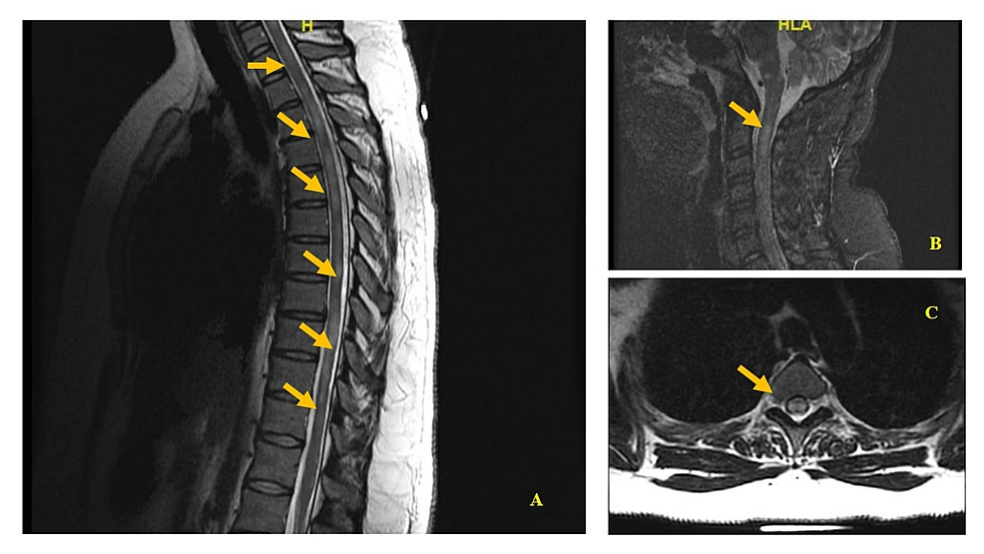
Magnetic resonance imaging of the thoracic spine. (A) and (C) show a plane image of the central spinal cord with a large ill-defined patchy hyperintense signal. (B) Axial T2-weighted cut (arrow) showing a slight expansion of the spinal cord diameter and hyperintense signal without pathologic contrast enhancement. The presence of hyperintensity in the spinal cord could indicate transverse myelitis (arrows).
